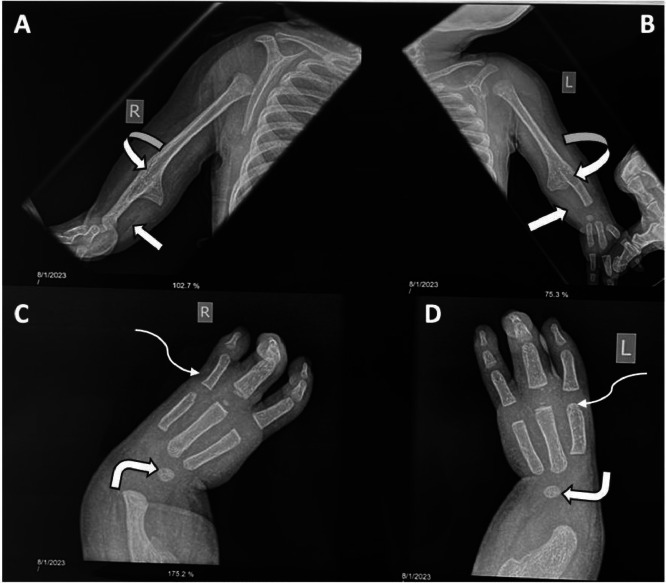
(A-D): Antero-posterior radiographs of both upper limbs (A and B) showing absence of the ulna bilaterally (block arrows) with elbow fixed in extension and bilateral humeroradial bony fusion (curved arrows). Anteroposterior radiographs of both wrists (C and D) show ulnar deviation with the presence of one carpal bone (bent arrows) and three digits (tridactyly) (squiggle arrows).
